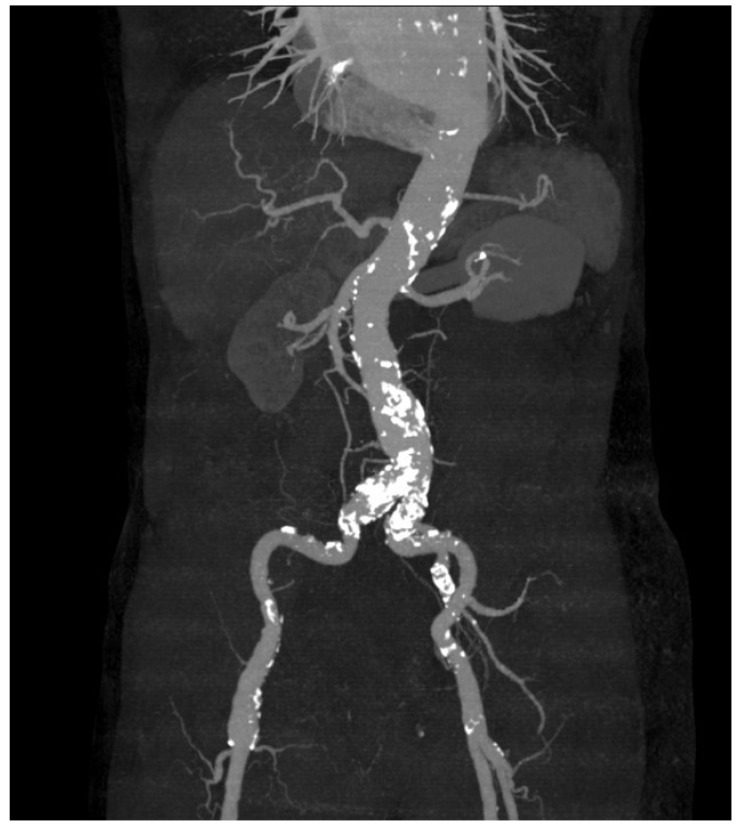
Post-operative computed tomography findings.
